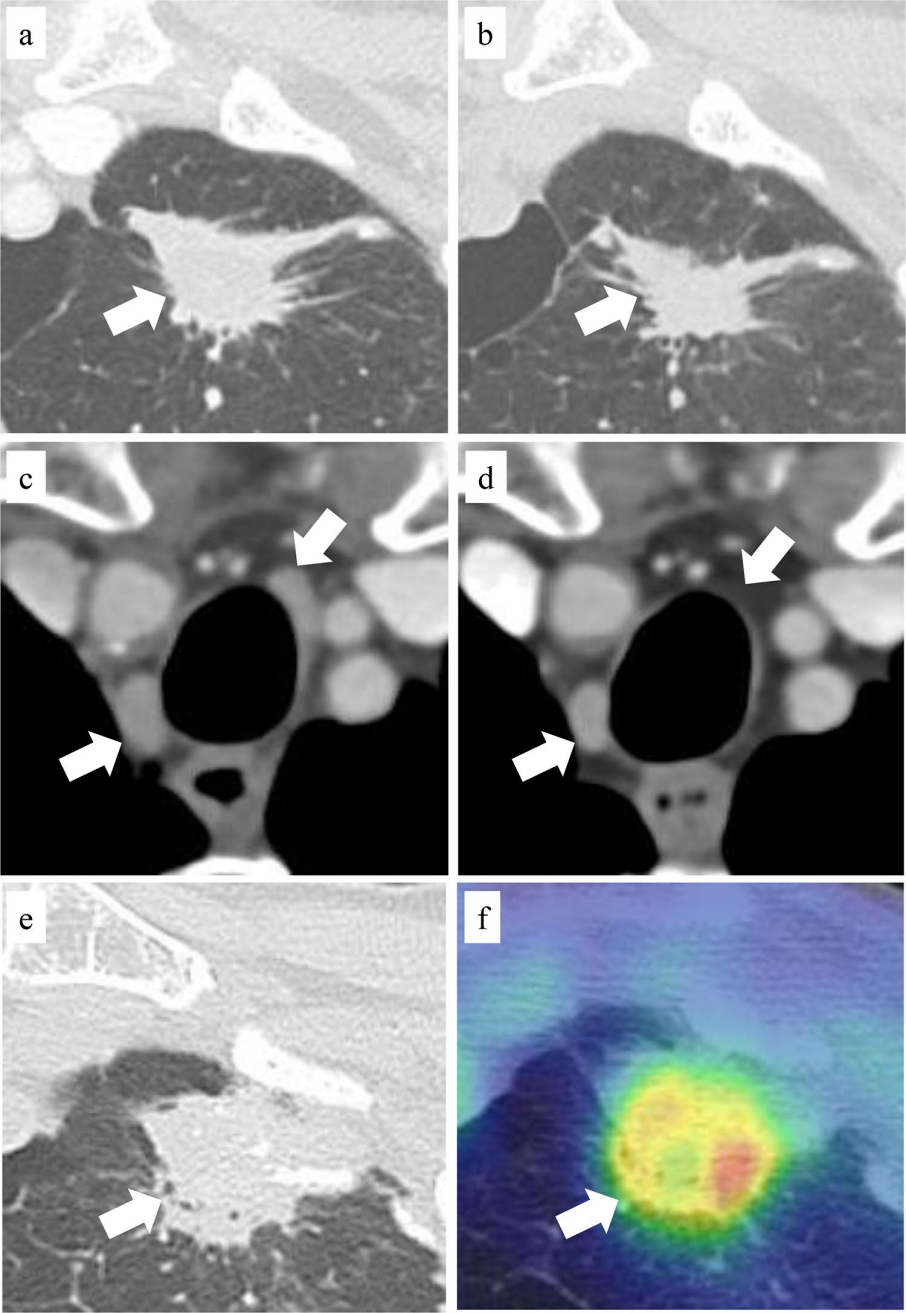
CT and PET/CT images of case 2. a Left primary lung lesion before radiotherapy; b left primary lung lesion 3 months after radiotherapy; c mediastinal lymph node before radiotherapy (unirradiated assessment lesion); d mediastinal lymph node 6 months after radiotherapy (unirradiated assessment lesion); e left primary lung lesion 1 year and 10 months after radiotherapy; and f left primary lung lesion 1 year and 10 months after radiotherapy (PET/CT). White arrow, tumour; *CT* computed tomography, *PET* positron emission tomography
